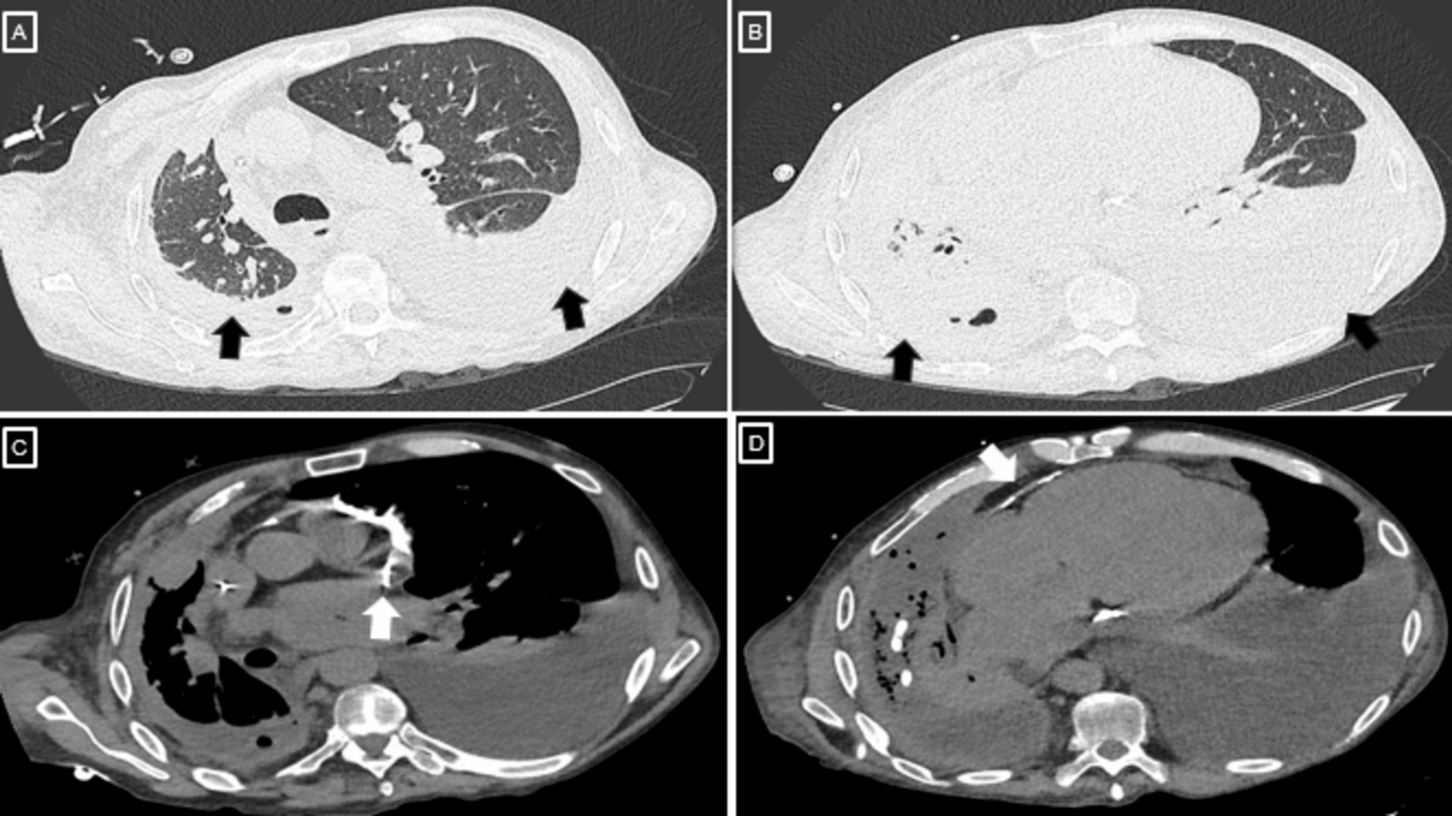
Chest computed tomography (CT) images (A, B) Axial CT views showing bilateral pleural effusions (black arrows). (C, D) Axial CT views demonstrating diffuse pericardial calcifications (white arrows) and cardiomegaly.
